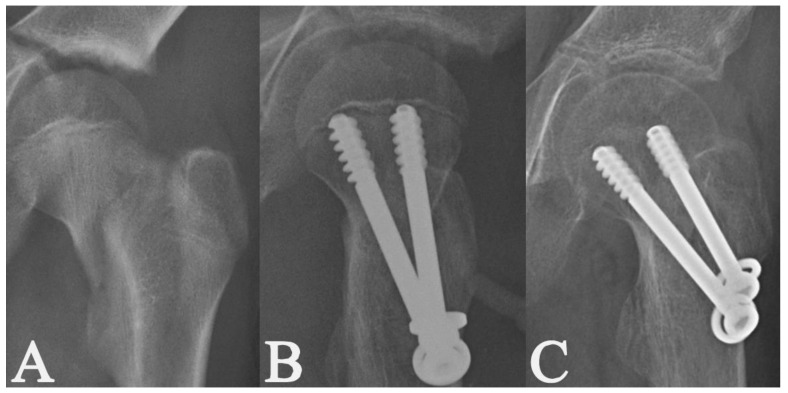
Radiographs of a 12-year-old boy with a displaced Delbet–Colonna type III fracture [15] (A); radiographs after open reduction and internal fixation with 2 cannulated ˅-shape screws; the fracture was completely compressed by the screws with the threads above the fracture line (B); the radiological healing at 3.5 months post-surgery (C).
